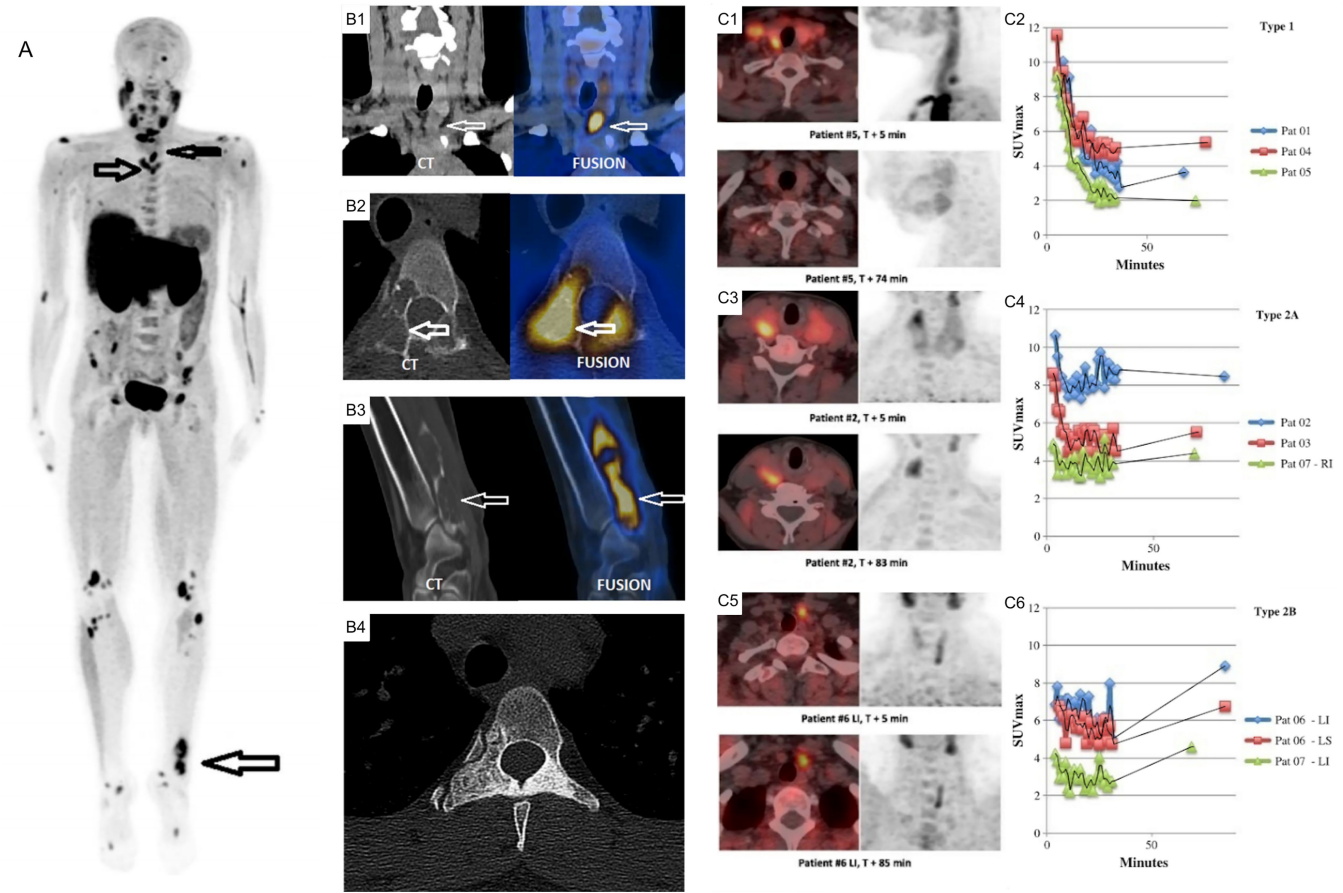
(A) 18F-FCH PET/CT whole body imaging in a patient with severe hyperparathyroidism depicting multiple brown tumors on maximum intensity projection (MIP) images (arrows), coronal CT (left) and fusion PET/CT (right) images depicting parathyroid adenoma (B1), axial (B2) and oblique (B3) CT (left) and fusion PET/CT (right) images depicting brown tumor (arrows), axial CT depicting sclerotic healed brown tumor (B4) following cure of hyperparathyroidism. (C) Comparison of acquisition protocols for 18F-FCH PET/CT showing cases where an earlier <10 min time point had higher uptake compared to a delayed >60min time point (C1, C2), cases where earlier and delayed time points where similar (C3, C4), and cases where the delayed time point had increased uptake (C5, C6). 18F-FCH, 18F-fluorocholine. Reproduced with permission from: (A, B)
